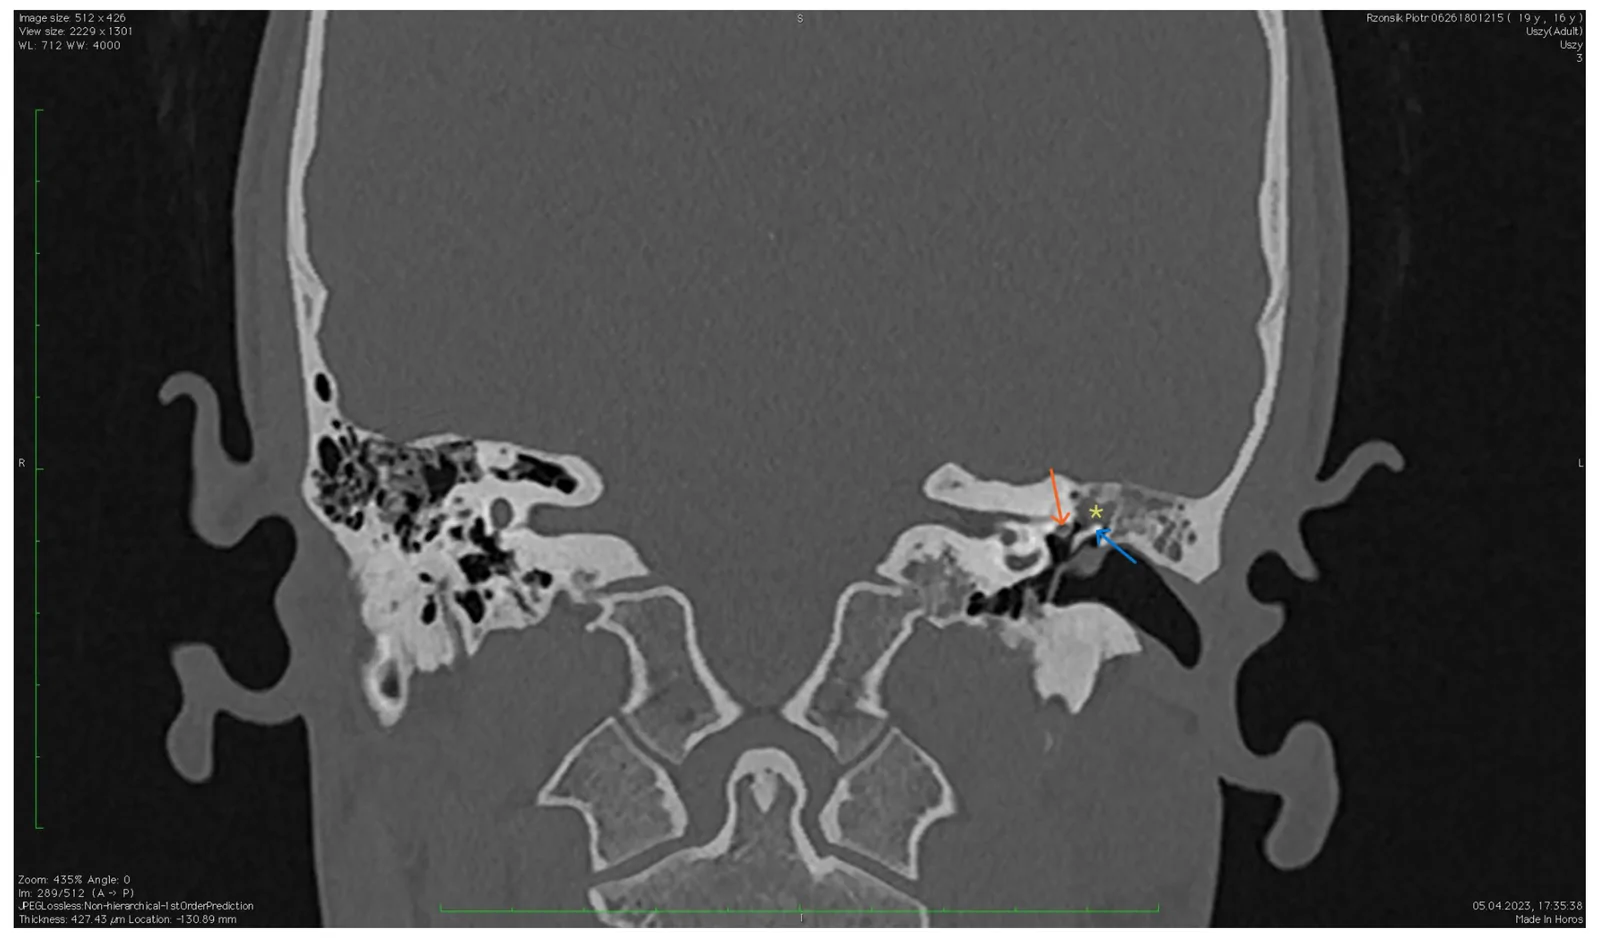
Pre-operative CT (left ear). The image shows a tumour involving the tympanic membrane, attic, and mastoid antrum. *—tumour; blue arrow—head of the malleus; orange arrow—facial nerve.
